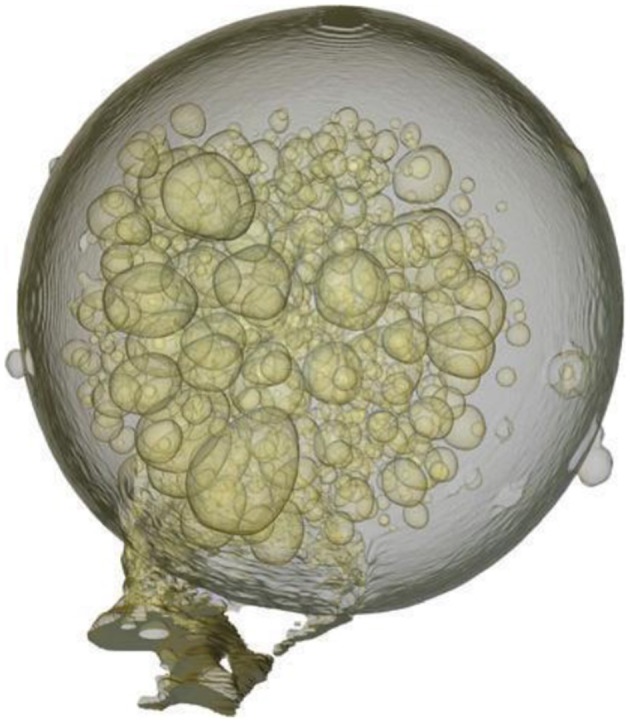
X-ray micro-tomography image of a Ceramisphere (a trade mark of Ceramisphere Pty Ltd) containing encapsulated limonene clearly seen as liquid bubbles. (Ciriminna and Pagliaro, 2013) Scheme also describes the general mechanism of encapsulation in glass shown as below.
